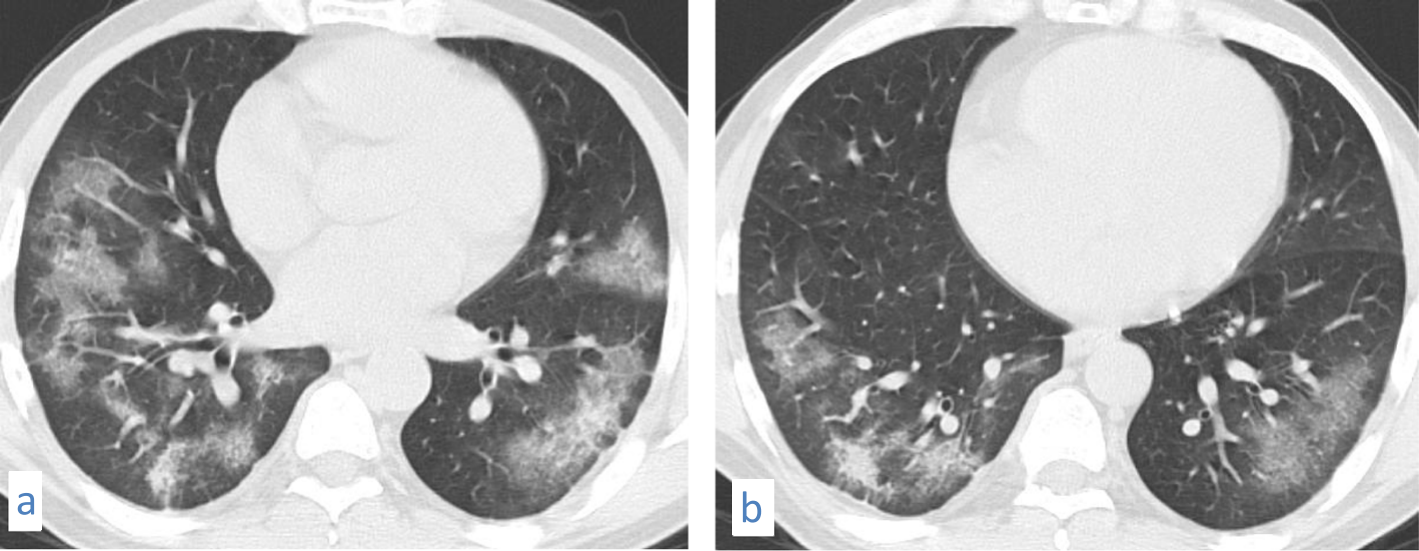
A 37-year-old male patient with COVID-19, CT chest axial images (a) and (b) show predominantly peripheral crazy paving with vascular thickening and traction bronchiectasis within. The affection is mostly in both lower lobes. A right peripheral basal patch of consolidation is noted
